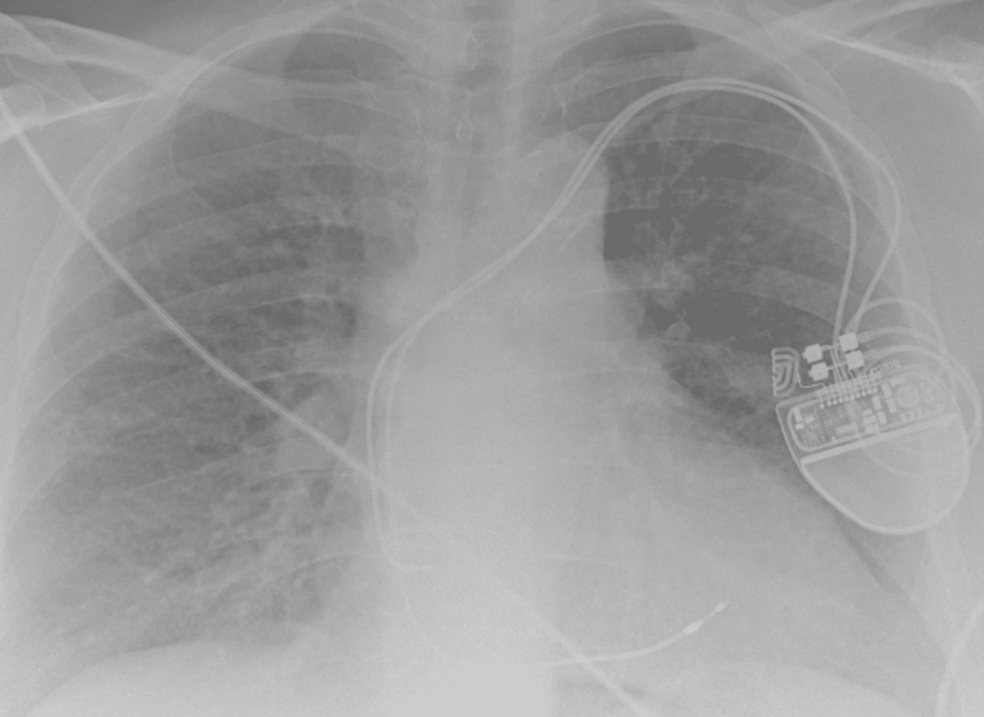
Chest radiography shows dual chamber pacemaker in situ
